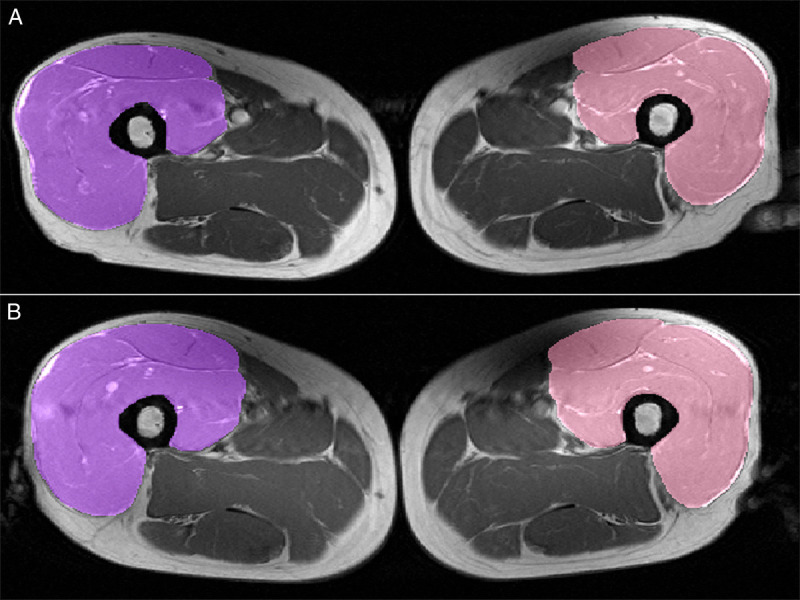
Muscle segmentation in MR images before and after training intervention. T1-weighted MR images with segmentation of the quadriceps femoris muscle (patient’s right side in purple and left side in pink) at baseline (a; top) and after the exercise training intervention (b; bottom). The femur, fatty, and connective tissues as well as vessels were excluded from the segmentation, but fascia lata and beginning rectus tendon were included. This technique and the slice-selection have been shown to adequately represent changes in extensor muscle volume (*r*^2^ = 0.73) (34).
